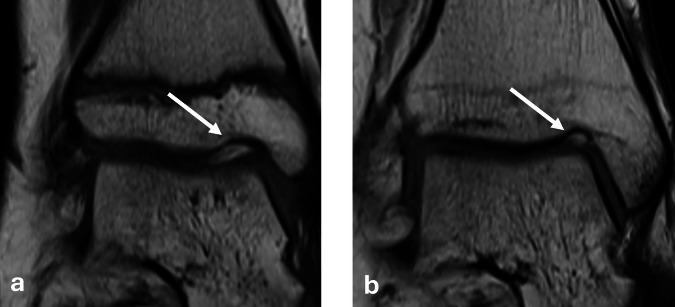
Coronal TSE MR images of the right ankle in a 13-year-old female patient at baseline (a) and after 7 years (b). White arrows indicate the Notch of Harty. The follow-up shows a clear morphological transformation over time, providing visual support for an age-related remodeling process
